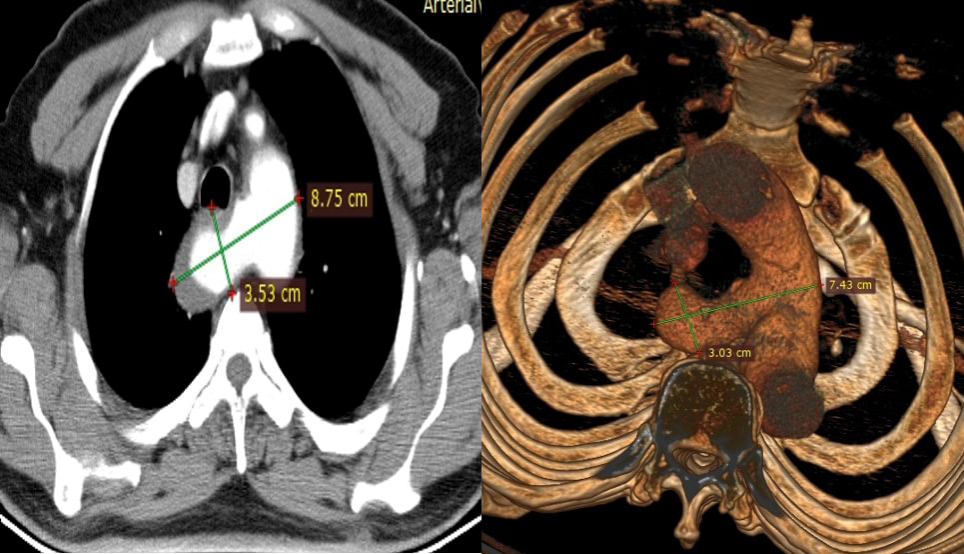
Thorax CT section and 3D image of the ARSA.
